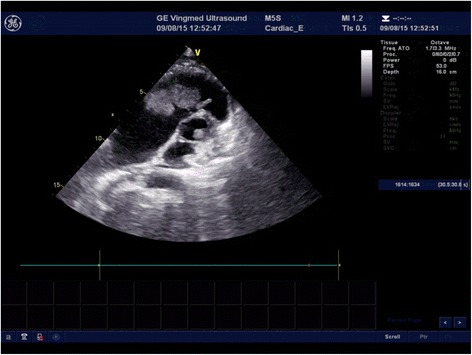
Transthoracic echocardiogram showing *right* and *left* ventricular mass
